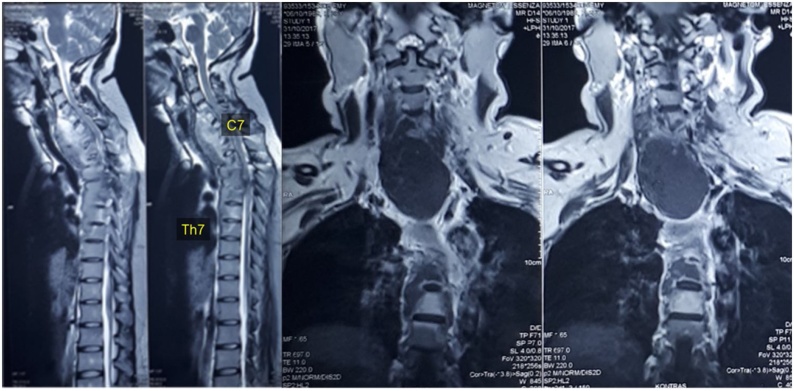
Sagittal cervicothoracic MR images demonstrated pathologic contrast enhancement on C4 to T7 segments along with vertebral body involvement and spinal canal compression at the level of C4 to T4. From coronal MR images, a large paravertebral abscess was presented anteriorly.
